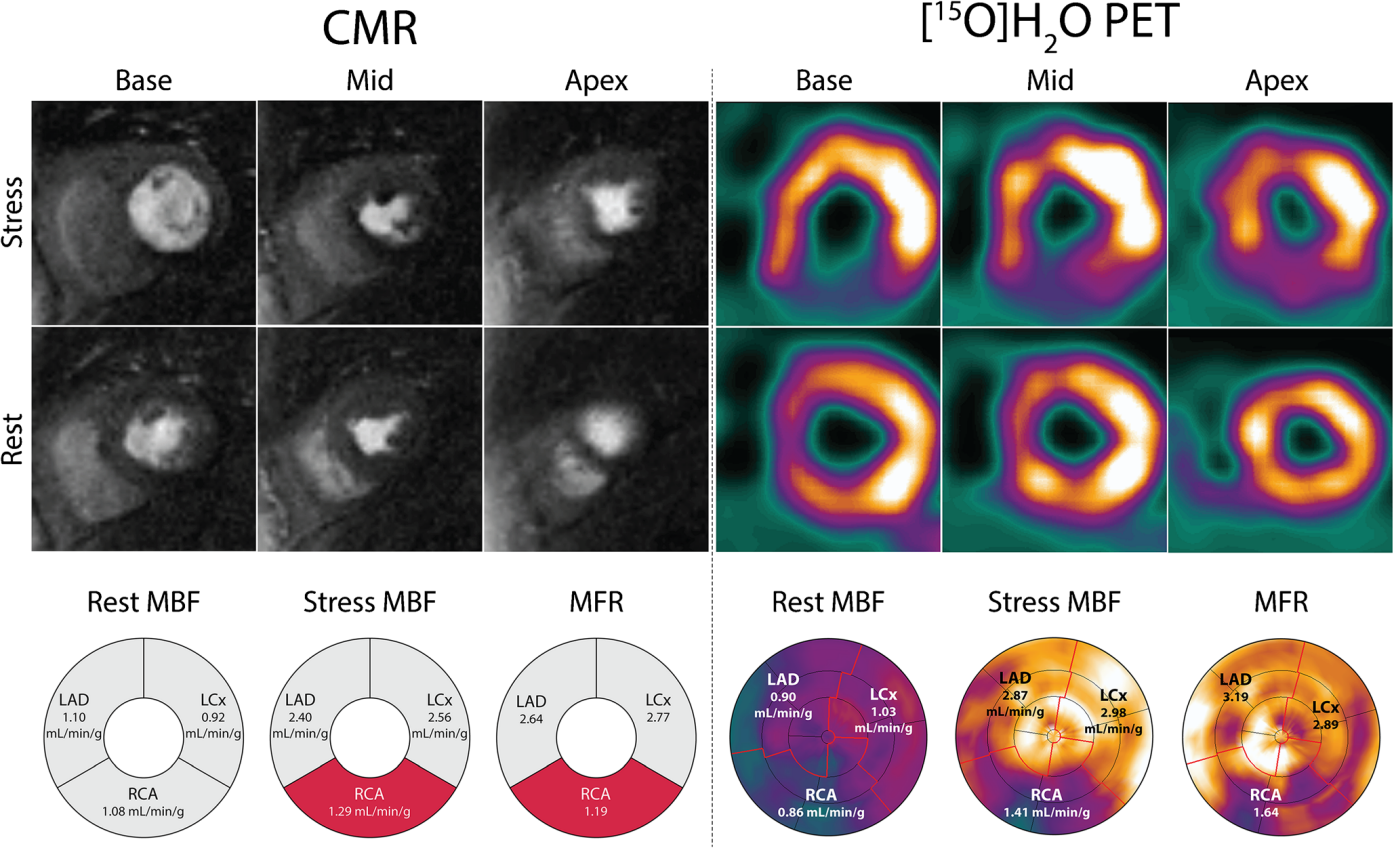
Case example of concordance between CMR and [^15^O]H_2_O PET in a 71-year-old female patient who presented with typical angina. Short-axis slices at the basal, mid, and apical levels have been selected from the PET study in order to match CMR and PET images. Both CMR and [^15^O]H_2_O PET demonstrate a perfusion defect in the inferior wall stretching from base to apex. With both techniques, the measured stress MBF and MFR in the vascular territory of the RCA are well below the ischemic thresholds. CMR = cardiac magnetic resonance imaging; LAD = left anterior descending artery; LCx = left circumflex artery; MBF = myocardial blood flow; MFR = myocardial flow reserve; PET = positron emission tomography; RCA = right coronary artery
